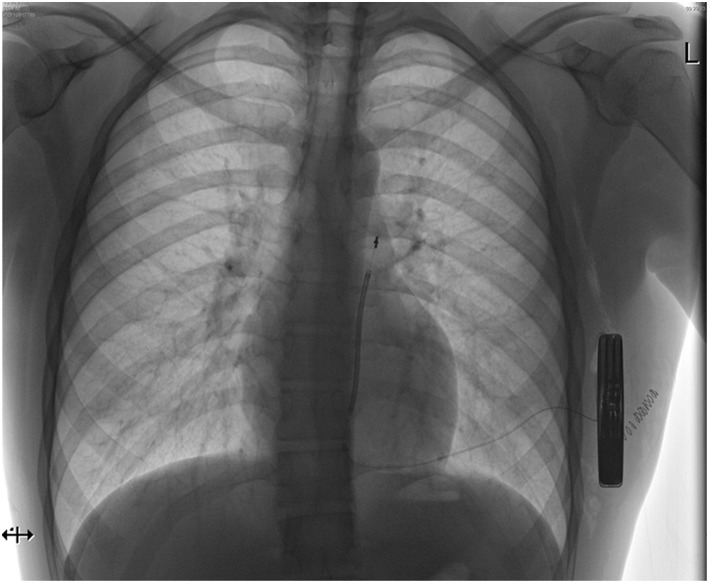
Antero-posterior chest X-ray after subcutaneous ICD (S-ICD) implantation.
